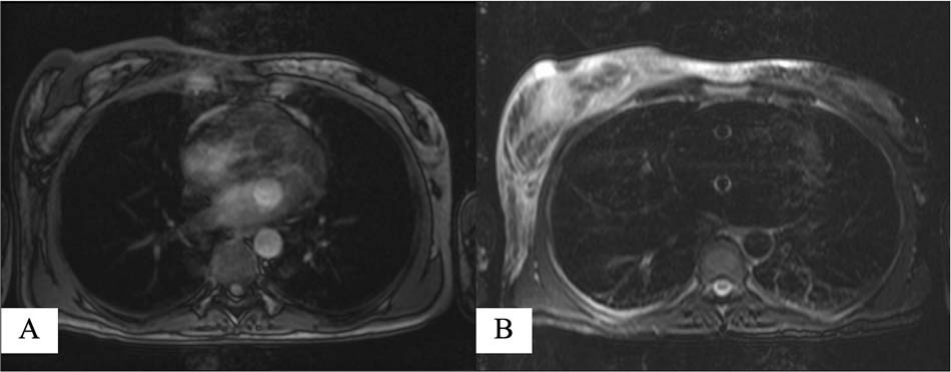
Magnetic resonance imaging reporting an area of 9.6 × 5 × 7.3 mm in the right breast enhanced by contrast uptake: (A) T1-weighted sequence and (B) T2-weighted sequence.
